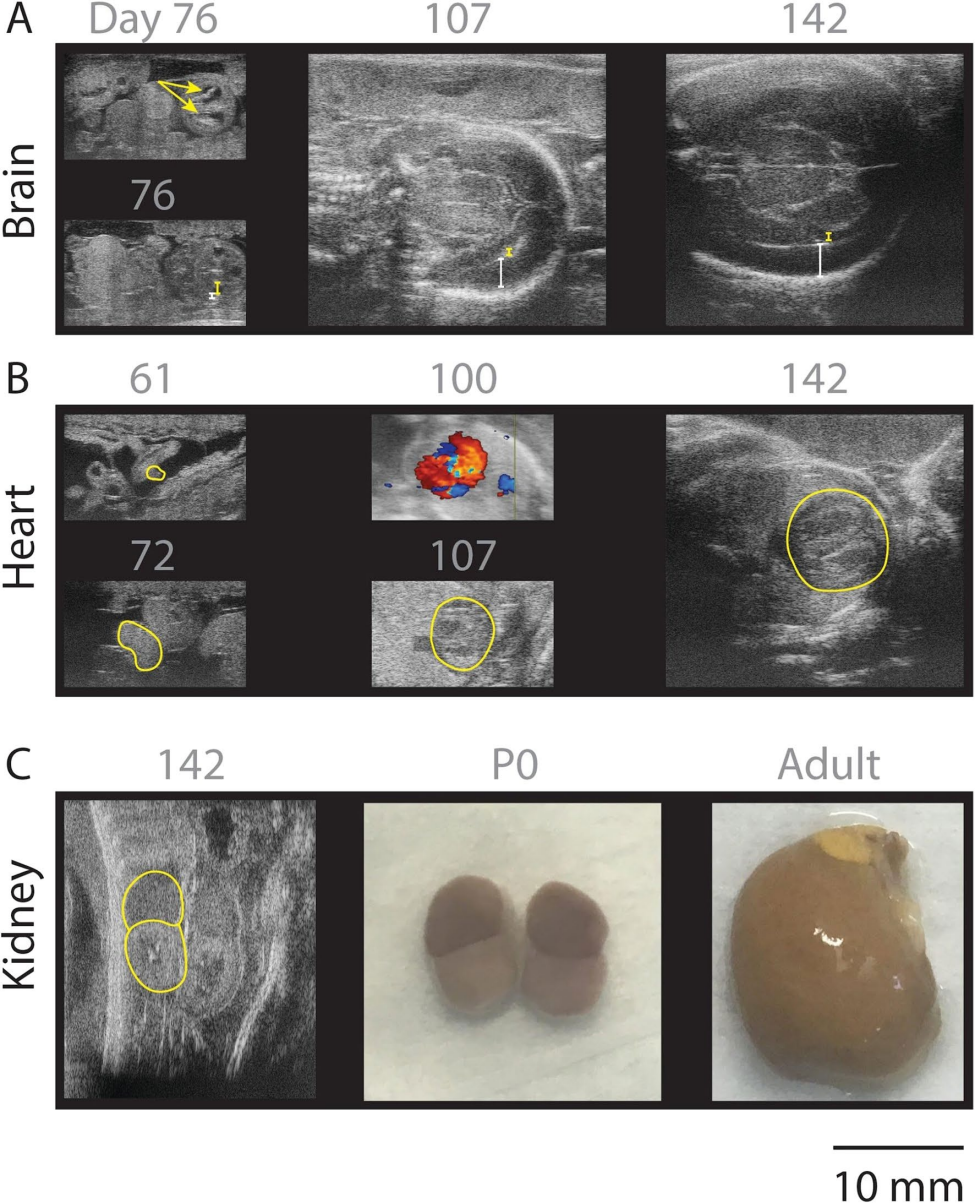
Organogenesis milestones tracked with ultrasound. A Brain development. Choroid plexus distinct within lateral ventricles as denoted by arrows. Large lateral ventricles (yellow bars) that gradually reduce in size as the cortical thickness increases over time (white bars). Days 76–107 are coronal views of the brain. Day 142 is a horizontal view. B Development of the heart tube with subsequent inclusion into the thoracic cavity and development of distinct chambers. Yellow outlines indicate regions of heart development. Day 100 is a Doppler scan of the heart in which blue and red indicate blood moving in different directions. C (i) Adrenal to kidney ratio on ultrasound reveals ratio of 1:1 shown to be accurate with (ii) ex vivo gross dissection. (iii) This ratio dramatically decreases as the kidneys and adrenals mature. All gray text indicates days postfertilization except for P0 which is day of birth and adult
